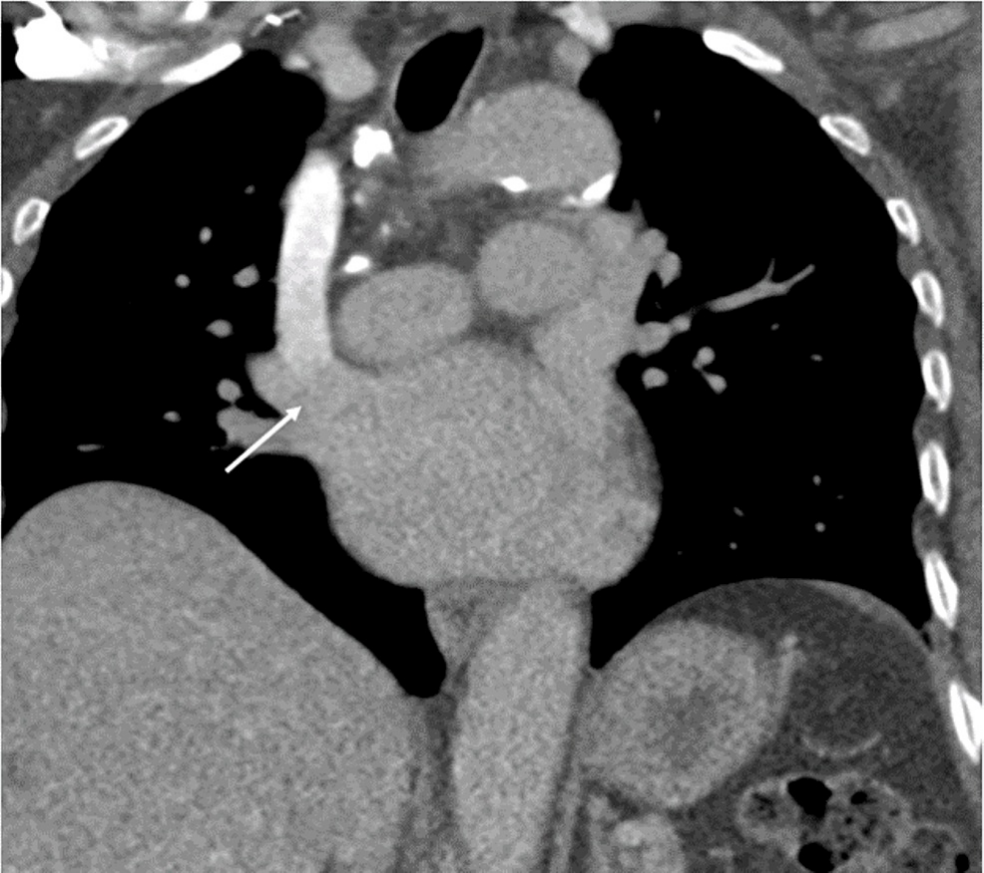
Coronal maximum intensity projection (MIP) image of the chest from the initial CT angiogram injected through the right upper extremity. There is no contrast within the vasculature of either lung. The contrast bolus is seen entering the heart directly from what is assumed to be a normal right-side SVC (white arrow). SVC: superior vena cava.
